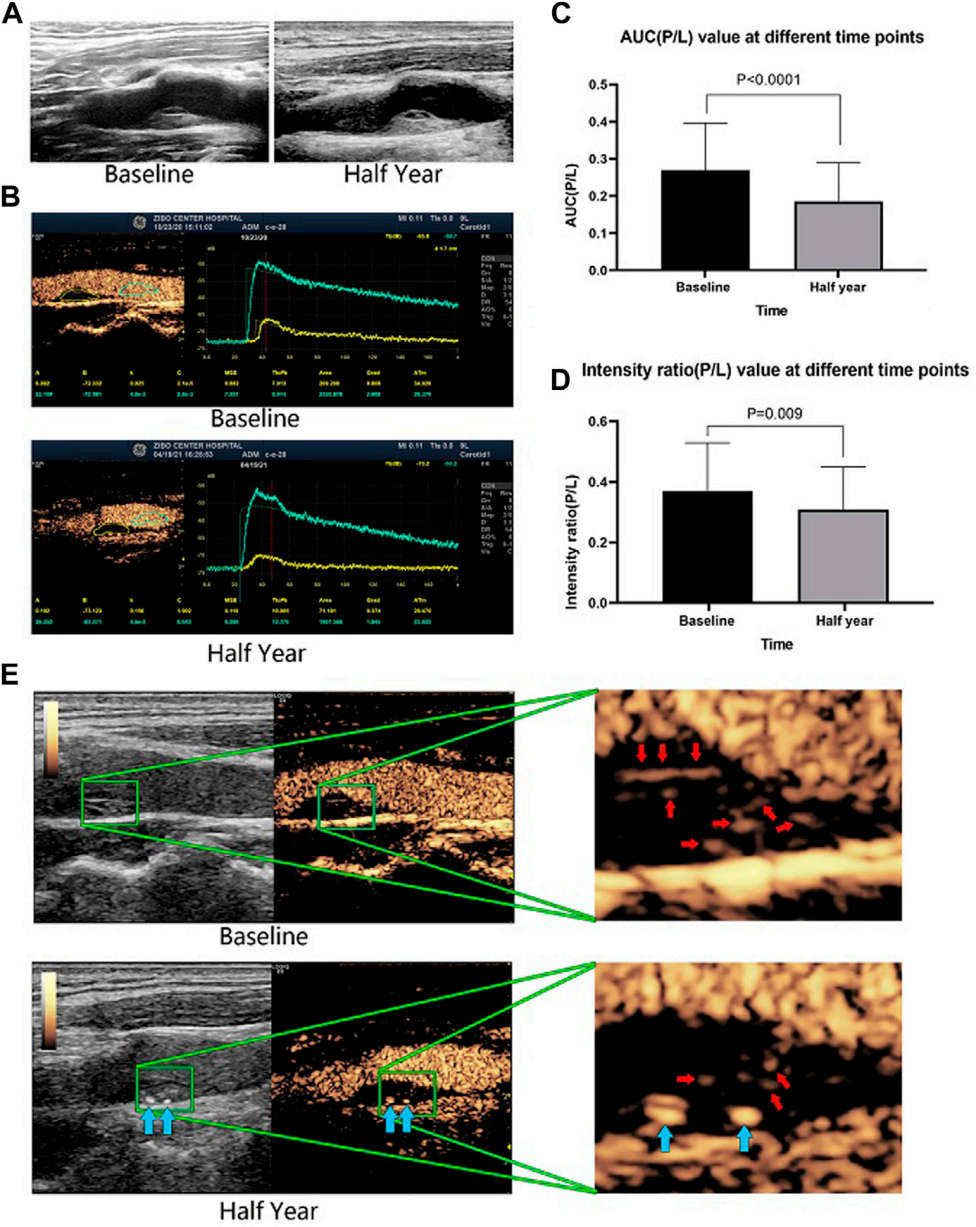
A representative patient who had a large plaque in the common carotid artery that was identified by B-mode ultrasound. The plaque morphology changed little between baseline and after half a year of therapy (A). CEUS revealed that the contrast agent area and microvessel density were obviously reduced after half a year of evolocumab plus statin treatment (B). The AUC (P/L) and the enhanced intensity (P/L) decreased significantly after half a year of treatment (E). Red arrows indicate vessels in the plaque, and blue arrows indicate calcified plaques. The changes in AUC (P/L) and enhanced intensity (P/L) of all included patients before and after half a year of treatment (C,D).
